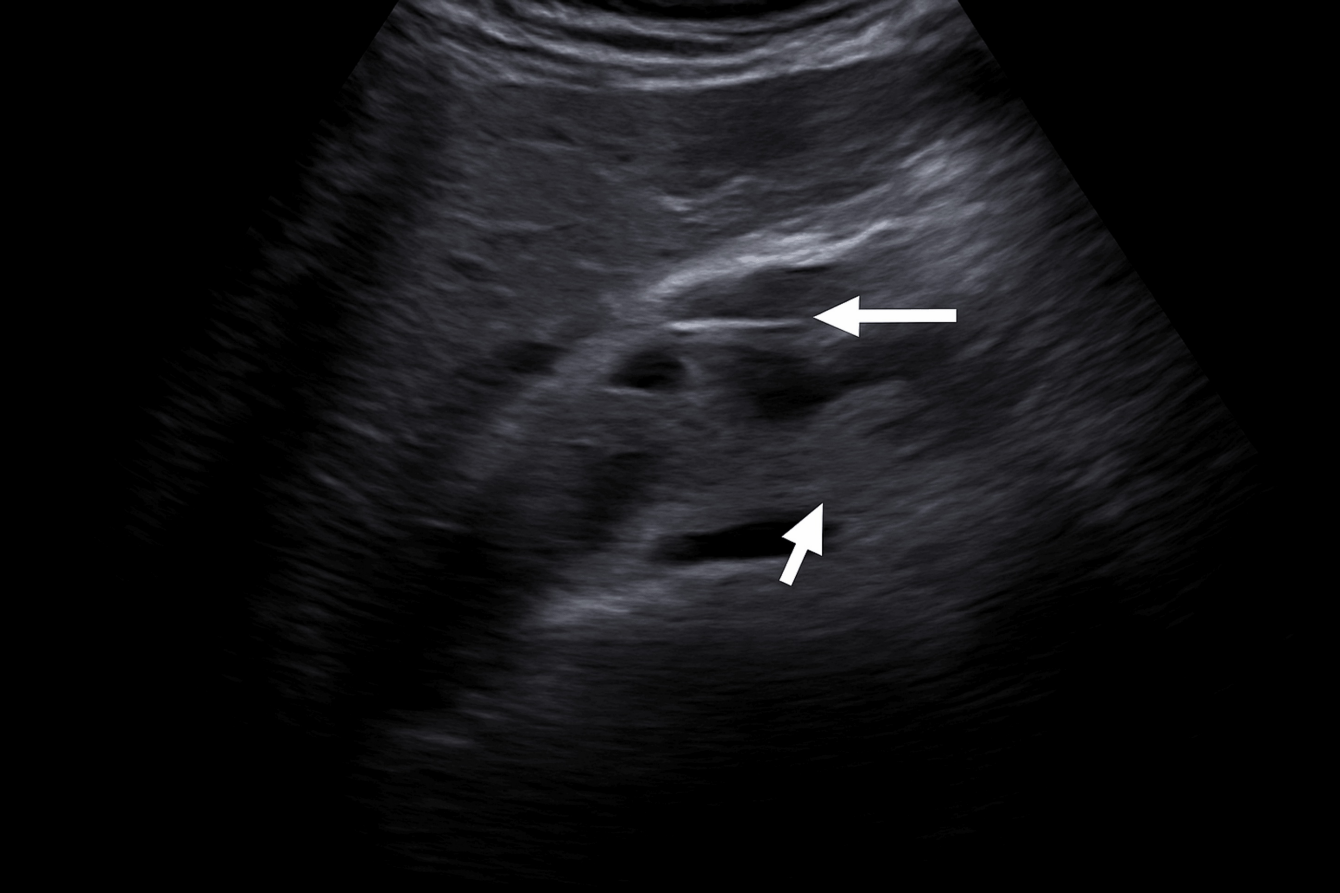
Doppler ultrasound image demonstrating the intrahepatic bile duct with a biliary catheter in situ (white arrow) The catheter appears as an echogenic linear structure within the common bile duct (CBD), confirming appropriate placement following endoscopic retrograde cholangiopancreatography (ERCP)
